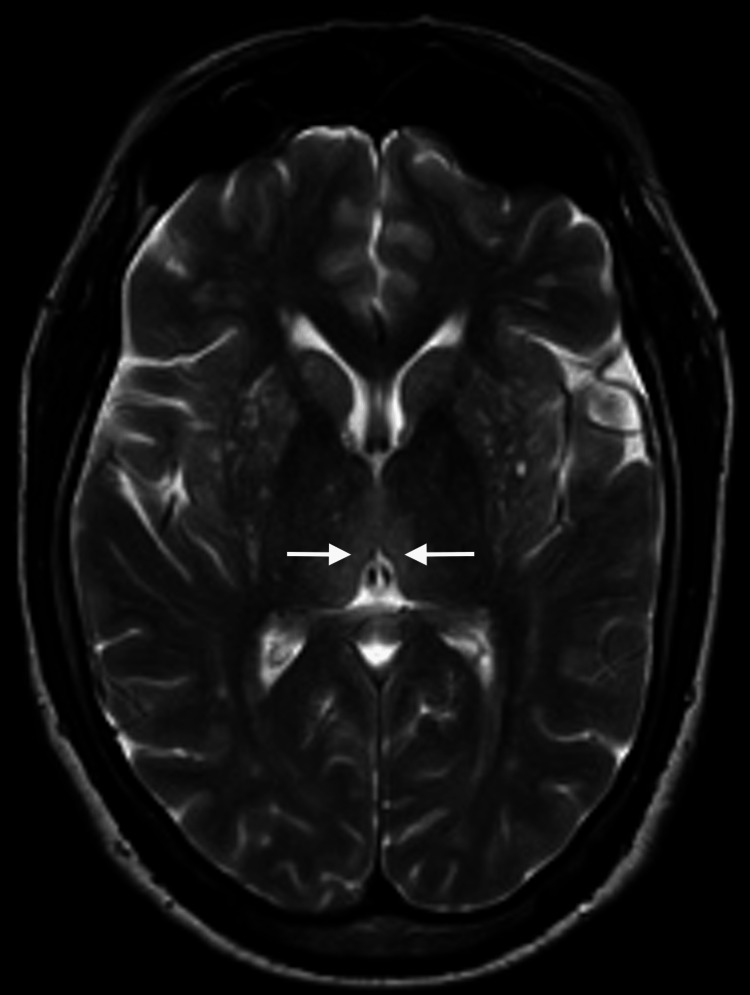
Contrast-enhanced MRI: Follow-up study. Axial T2 thalami follow-up. Slight improvement in bilateral and symmetrical hyperintensity of the dorsomedial aspect of the thalami is observed on T2-weighted images (arrows).
